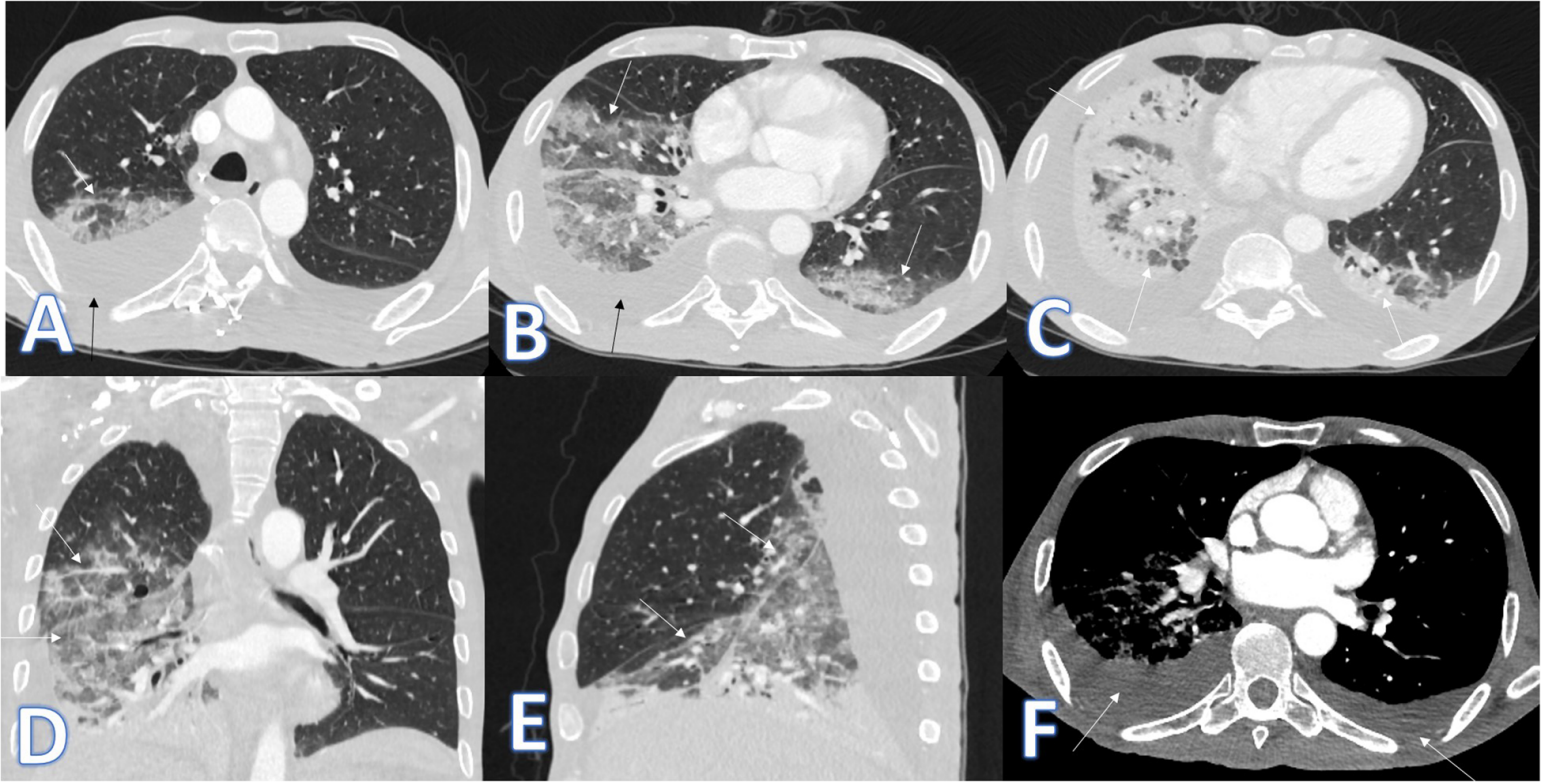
A 53-year-old male patient with cough and dyspnoea. CT showed multiple consolidative patches with ground glass appearance involving the whole right lower lobe, lateral segment of the middle lobe, and superior and posterior segments of the left lower lobe with bilateral pleural effusions (arrows). The diagnosis was mycoplasma pneumoniae
